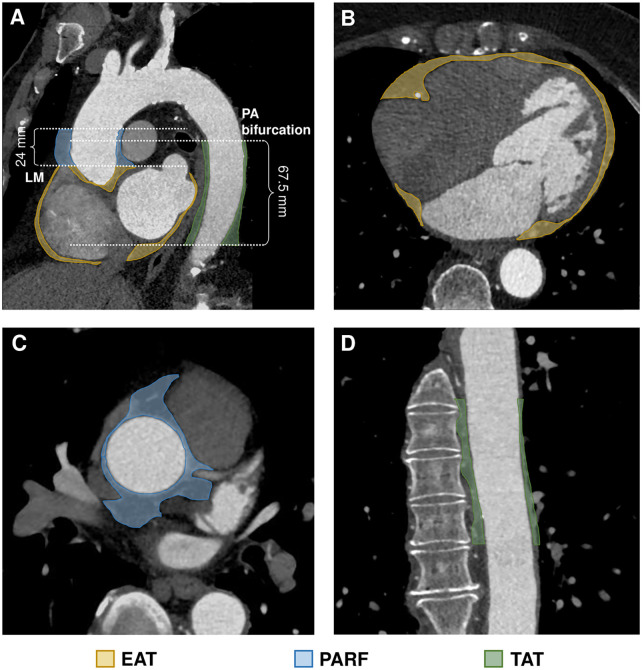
Measurement of intrathoracic visceral adiposity. Annotated CT images showing segmentation and quantification of epicardial adipose tissue [EAT; (B)], peri-aortic root fat [PARF; (C)], and thoracic peri-aortic adipose tissue [TAT; (D)]. Key landmarks include the pulmonary artery (PA) bifurcation and left main (LM) coronary artery (A).
